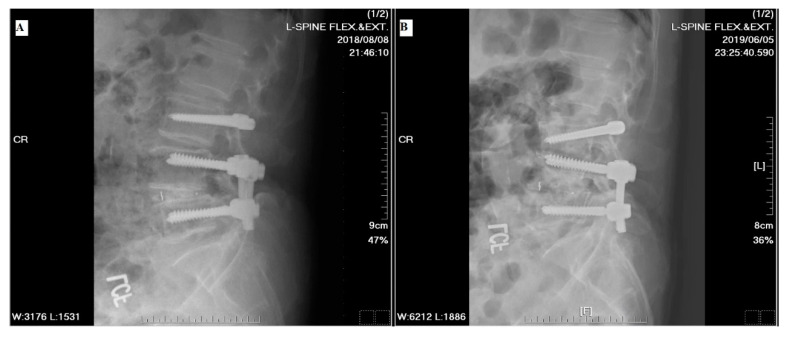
A 76-year-old male suffered from trauma-related implant loosening. (A) The 18-month postoperative radiographic follow up indicated the appropriate position of instrumentation. (B) The 24-month postoperative radiographic follow up revealed deviation in the bilateral L3, L4, and L5 screws after an automobile traffic accident.
